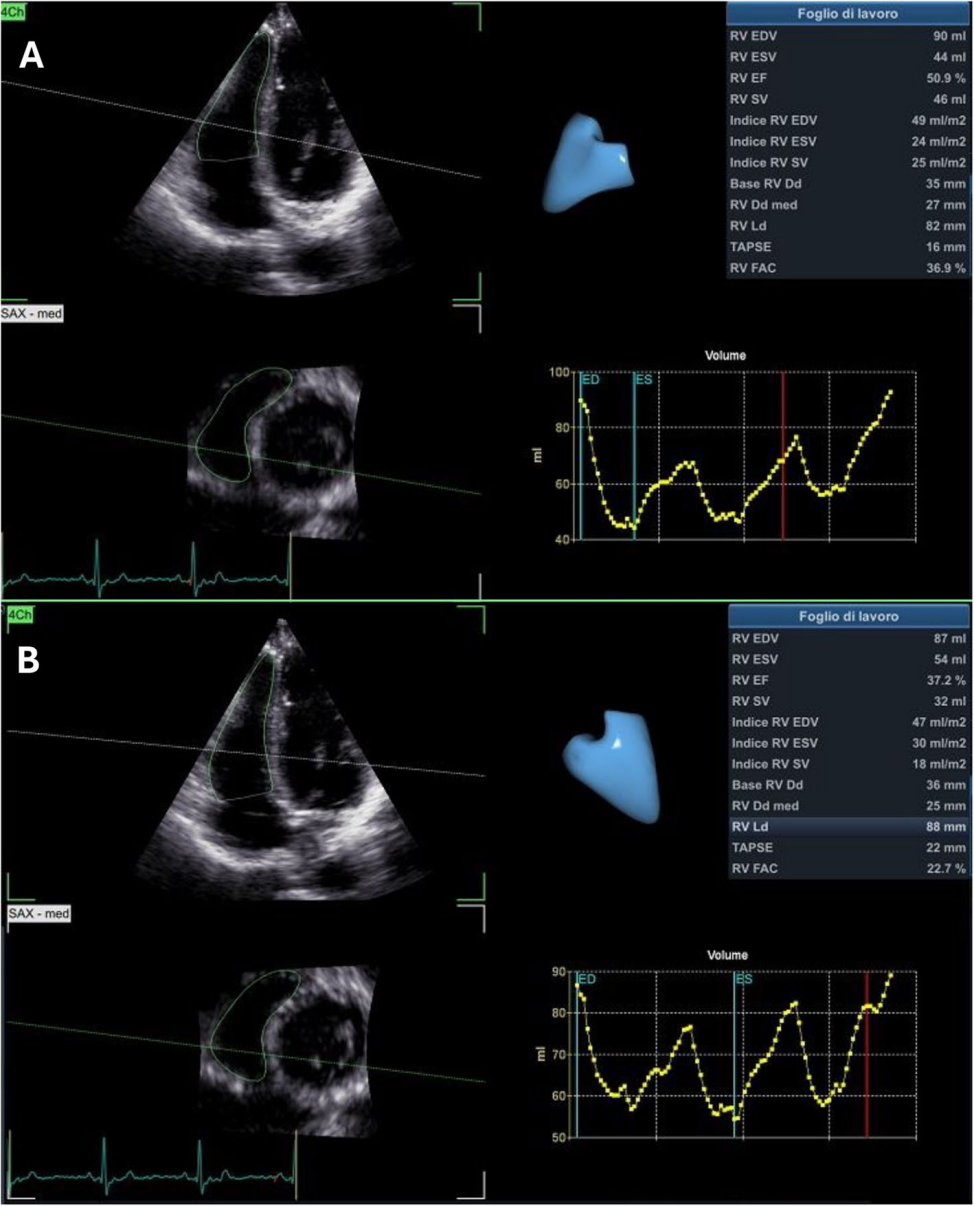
RV-3D echocardiographic evaluation of a patient before (A) and after (B) chemotherapy treatment
